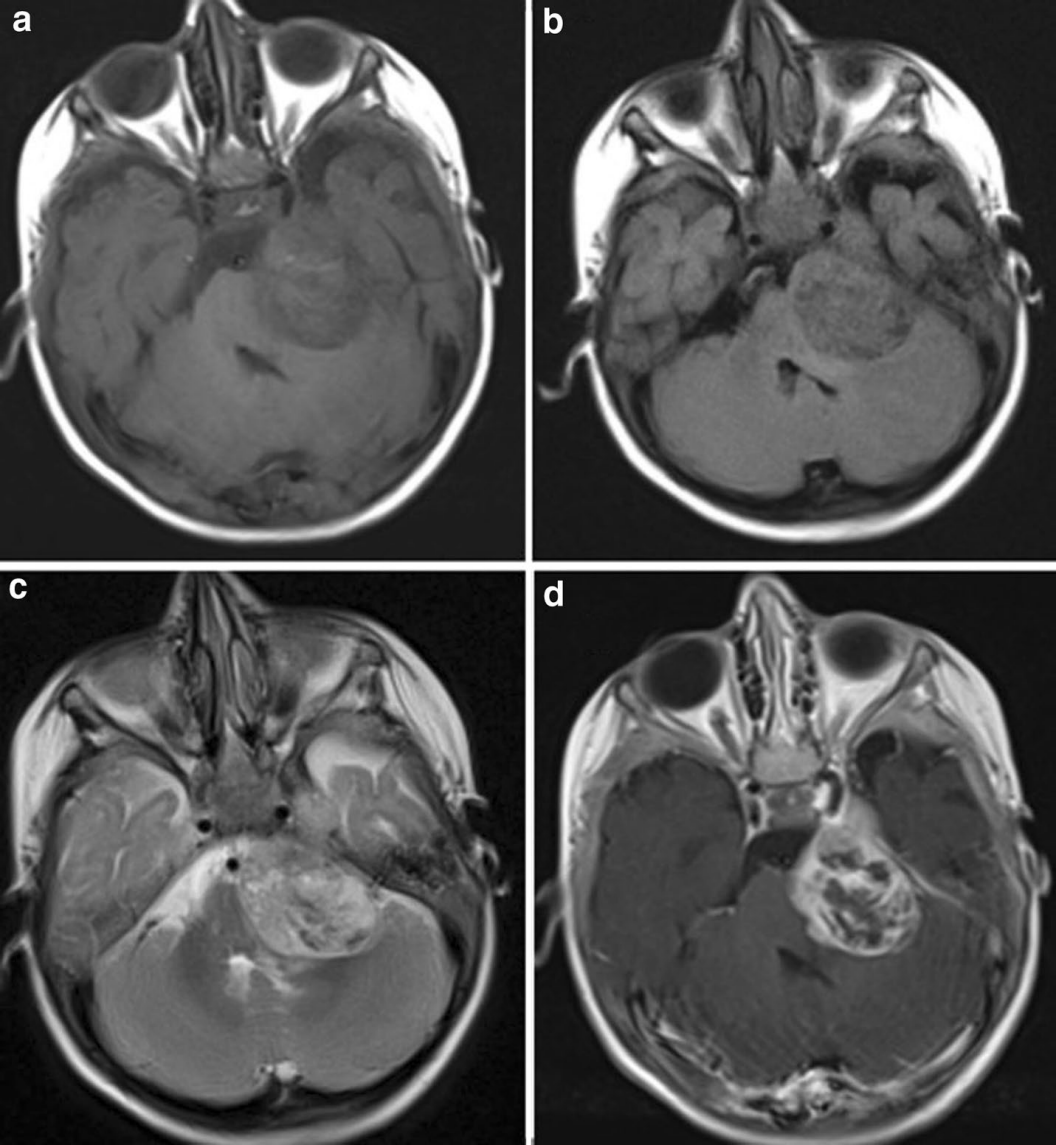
a–d On the brain MRI, a dural-based, extra-axial mass lesion was observed on the left CPA, 4 × 3 cm in size, with heterogenous contrast after the application of IV contrast dye, which was heterogenous-hypointense on T1W and FLAIR sequences, heterogenous hyperintense on T2W, the mass appears to enter the cavernous sinus via Meckle’s cave. Time there is concomitant calcification, bleeding and oedema of the surrounding
